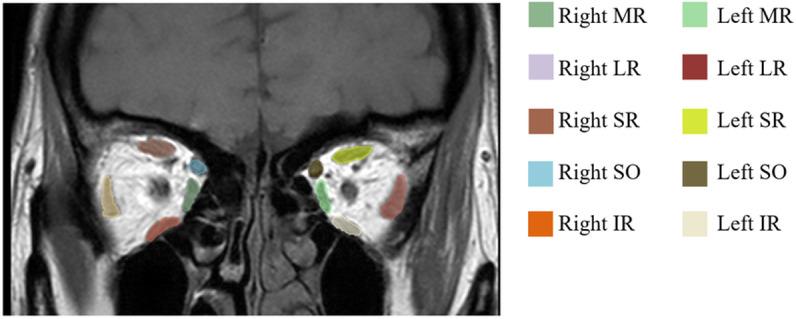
MRI T1 weighted images in coronal plane of a representative case with EOM segmentation by using 3D slicer software.
